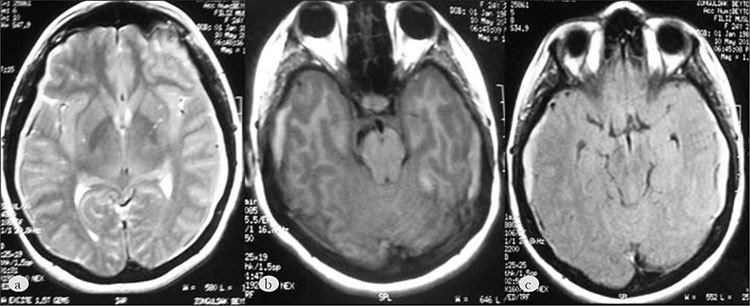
The cranial magnetic resonance imaging revealed bilateral subacute subdural haematomas in the frontotemporoparietalregion on axial T2-weighted image (a), T1-weighted (b), FLAIR image (c) respectively.
